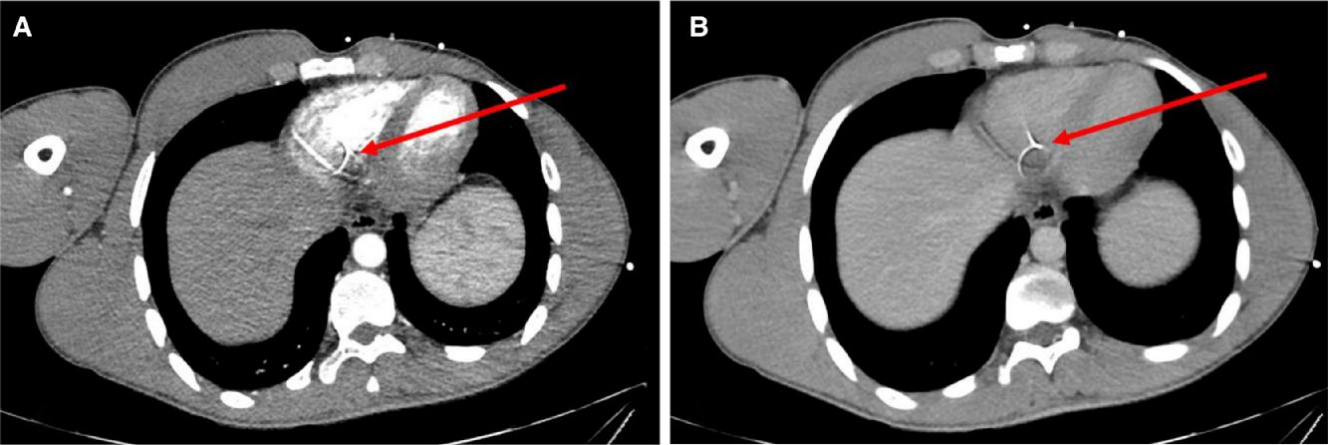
Axial images of arterial (*A*) and venous (*B*) phases of total body computed tomography performed at presentation showing the shrapnel in the right ventricle with adjacent metal artefacts.
